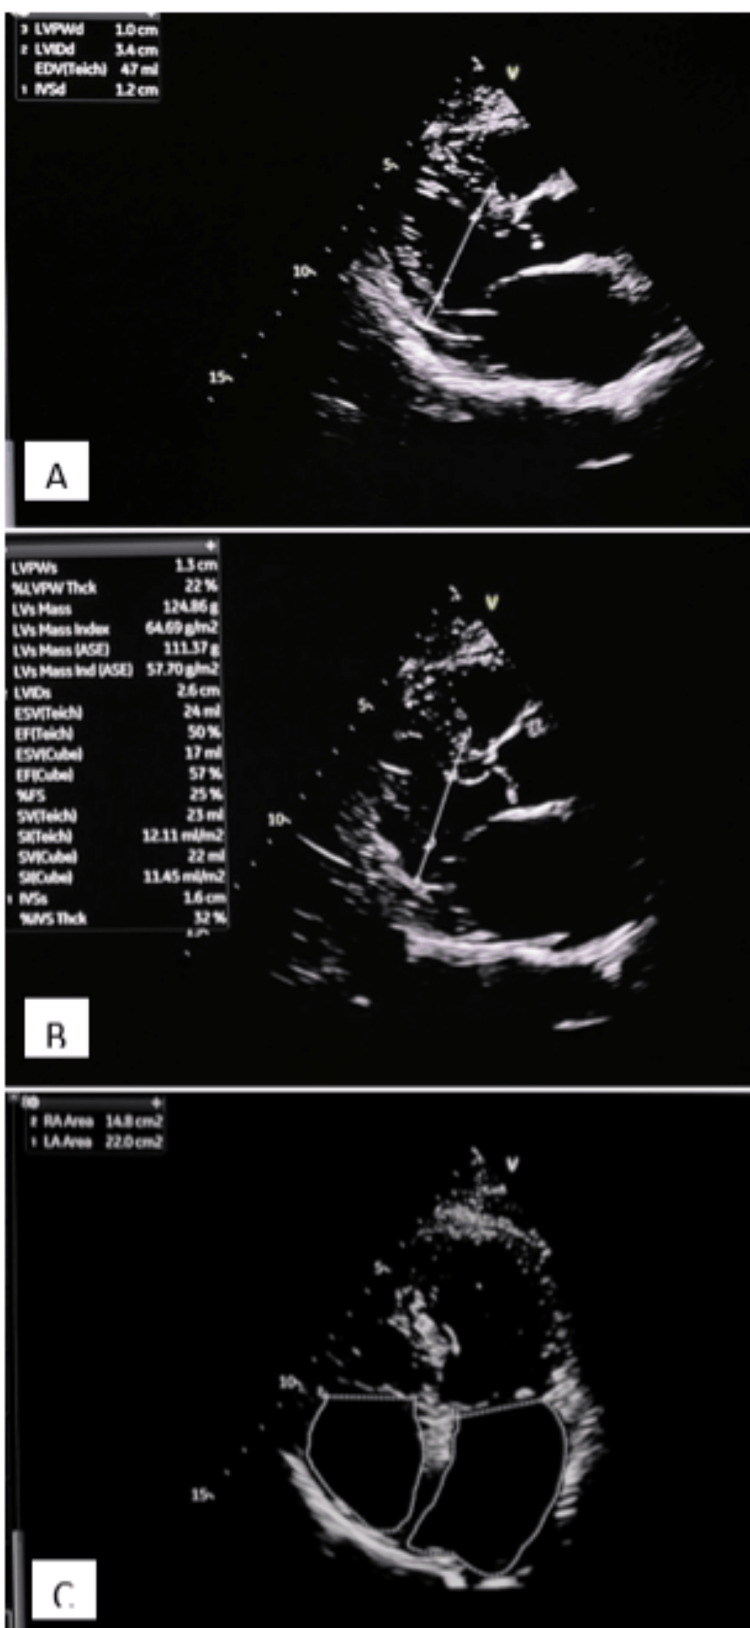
Echocardiographic images showing concentric left ventricular hypertrophy and dilated left atrium A: Parasternal long-axis view in systole, with EF of 50%. B: Parasternal long-axis view in diastole; the interventricular septal wall thickness in diastole measures 1.2 cm. C: Apical four-chamber view showing dilated left atrium; the left atrial area measures 22 cm^2^. EF: ejection fraction
